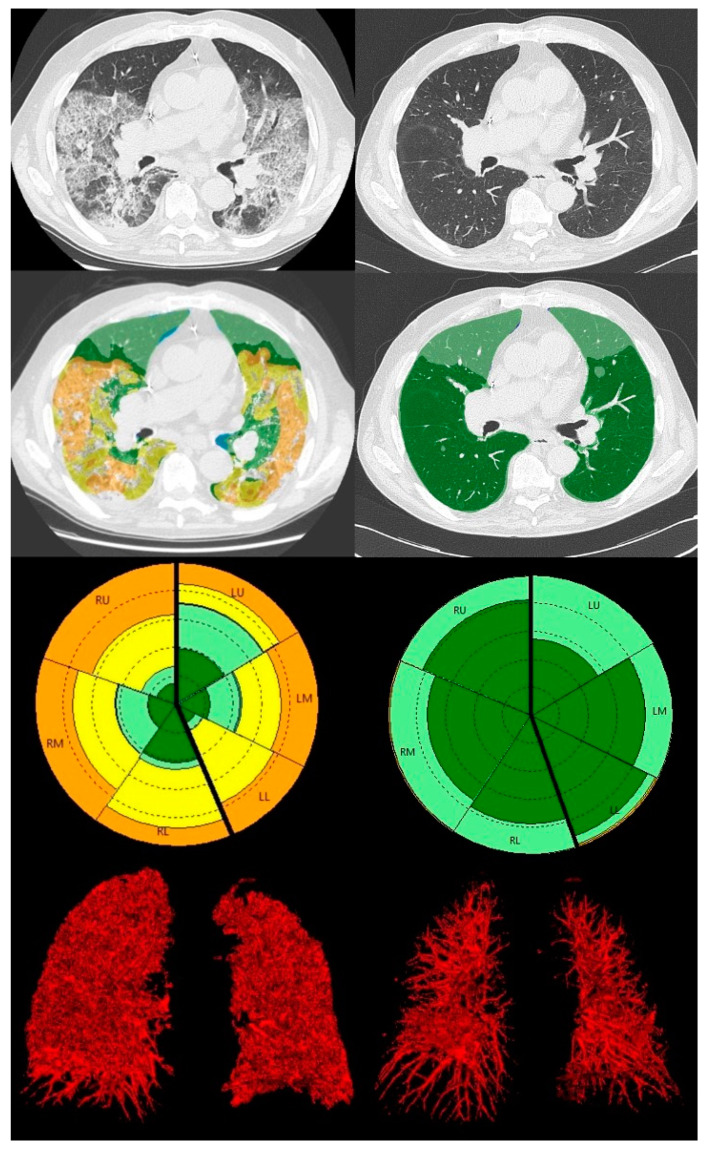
CALIPER analysis of a CT scan of an 84-year-old male patient at T0 (left) and T3 (right). From the upper to the bottom: axial CT slice, axial CALIPER-derived color image overlays (dark and light green = Norm, yellow = GG, orange Ret), the glyph of the lung parenchymal patterns and the 3D volume rendering reconstructions of lung vessels. Compared to the baseline, after three months, most of the abnormalities are resolved, both GG and Ret. A reduction in the VRS is also demonstrated in the 3D volume rendering.
